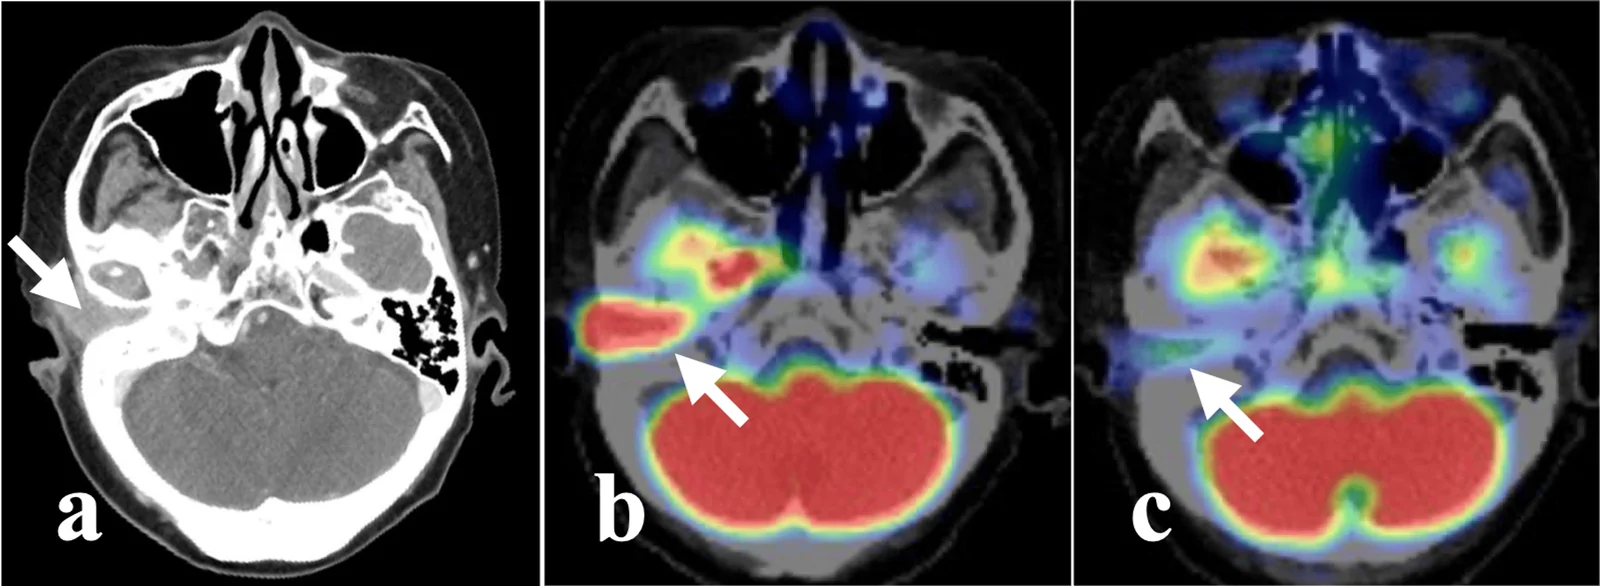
CT images of the patient showing right external auditory canal cancer (a, b) Pre-treatment images. (a) Contrast-enhanced axial CT showing an enhancing right external auditory canal lesion (white arrow); (b) Axial FDG-PET/CT demonstrating intense FDG uptake in the right external auditory canal lesion (white arrow); (c) Axial FDG-PET/CT obtained six months after CRT, showing complete regression of the right external auditory canal lesion and resolution of the abnormal FDG uptake (white arrow). CT; computed tomography, CRT; conventional radiotherapy, FDG; 2-deoxy-2-[¹⁸F]fluoro-D-glucose, PET; positron emission tomography.
